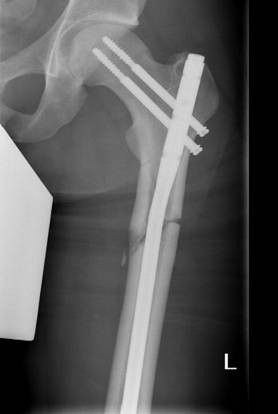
Post-operative radiograph showing antegrade intramedullary nailing of the left femoral shaft.
